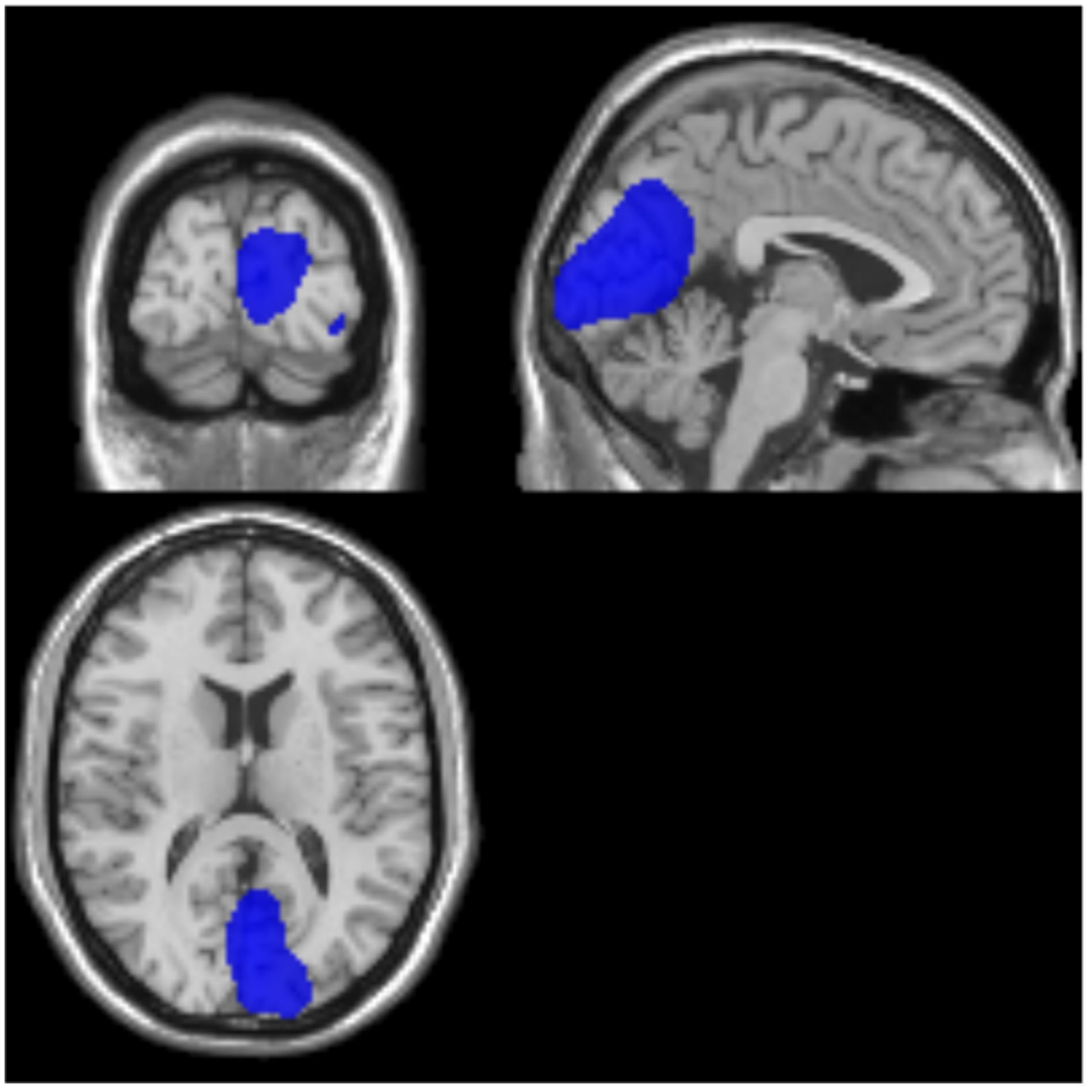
Examples of representative SUV for UWS/MCS. Blue color represents a high brain metabolism. Areas where activity in MCS is significantly higher than in *VS*/UWS (*p* < 0.001). MCS, minimally conscious state; UWS, unresponsive wakefulness syndrome.
